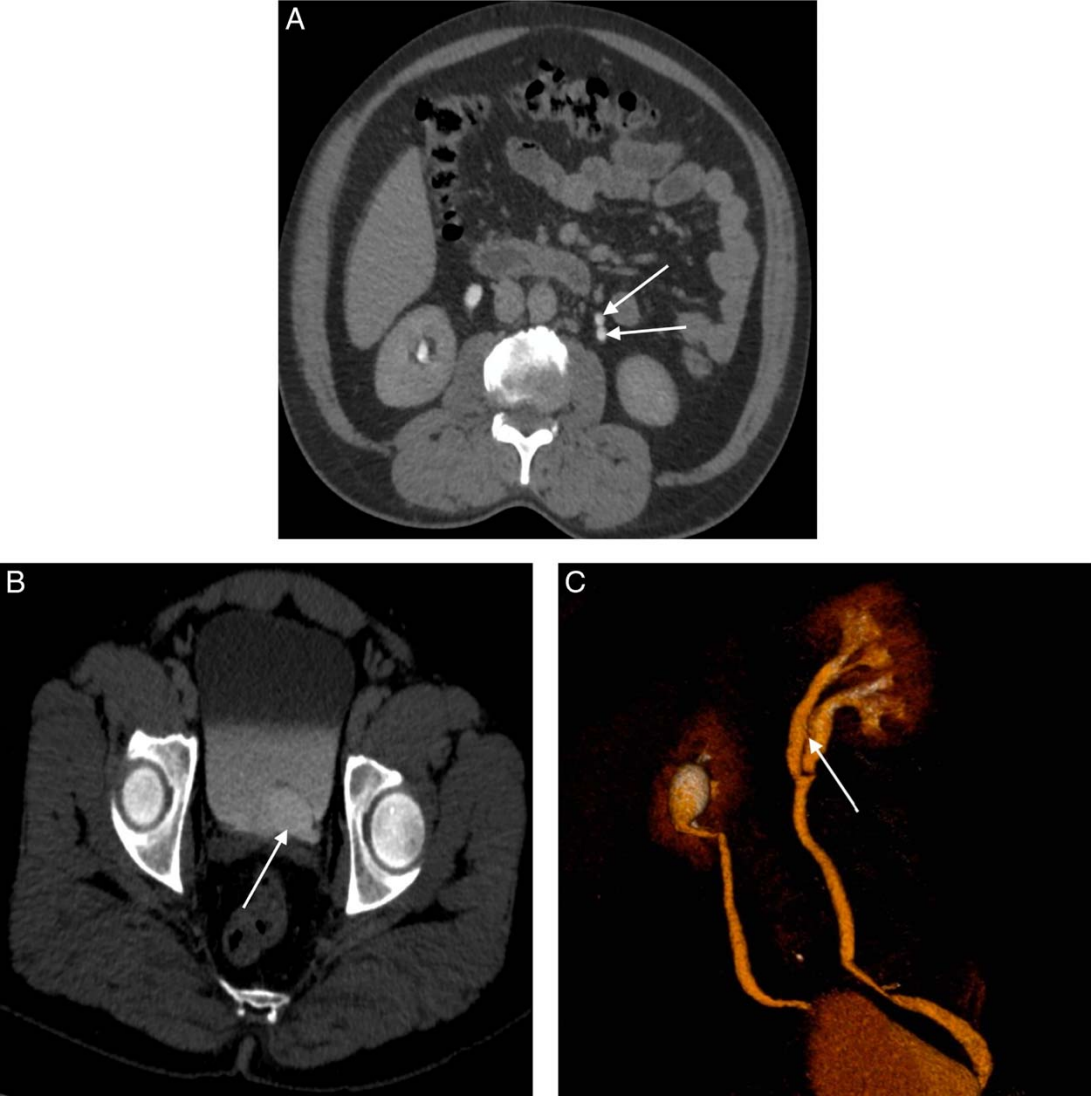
(A) Axial delayed phase contrast enhanced computed tomography (CT) image at lower level of kidney shows duplex collecting system in left side (white arrows). (B) Delayed phase axial contrast enhanced CT image at the level of pelvis shows the contrast filled outpouching (white arrow) arising from the left distal ureter. (C) 3D volume rendering technique of CT urography showing the partial duplex collecting system in left side (white arrow).
